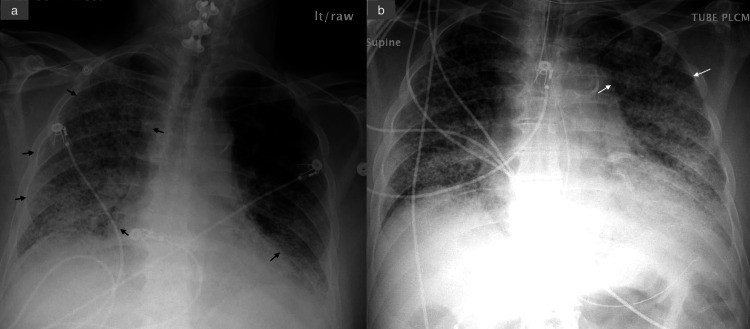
CXR showing progressive worsening of airspace opacities from admission to day 7 (post-intubation) (a) CXR on admission demonstrating a mixed reticular and consolidative pattern throughout both lungs (black arrow), more pronounced in the right hemithorax. (b) Repeat CXR on day 7, after intubation, showing diffusely worsened bilateral pulmonary infiltrates, with significant progression of airspace disease in the left upper lobe (white arrow). CXR, chest X-ray
